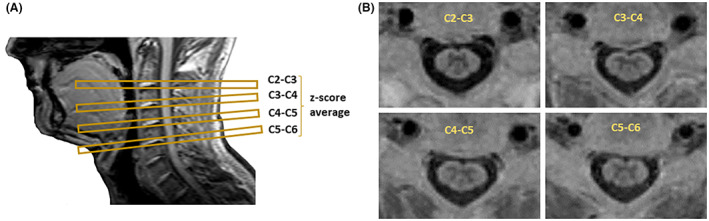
Example of PSIR magnetic resonance imaging acquisition. Panel A: axial 2D PSIR slices were acquired at four cervical segments (i.e., C2–C3, C3–C4, C4–C5, and C5–C6) perpendicular to the long axis of the cervical cord and centered at each intervertebral disc. Panel B: phase‐sensitive reconstructed PSIR images for each cervical segment.
